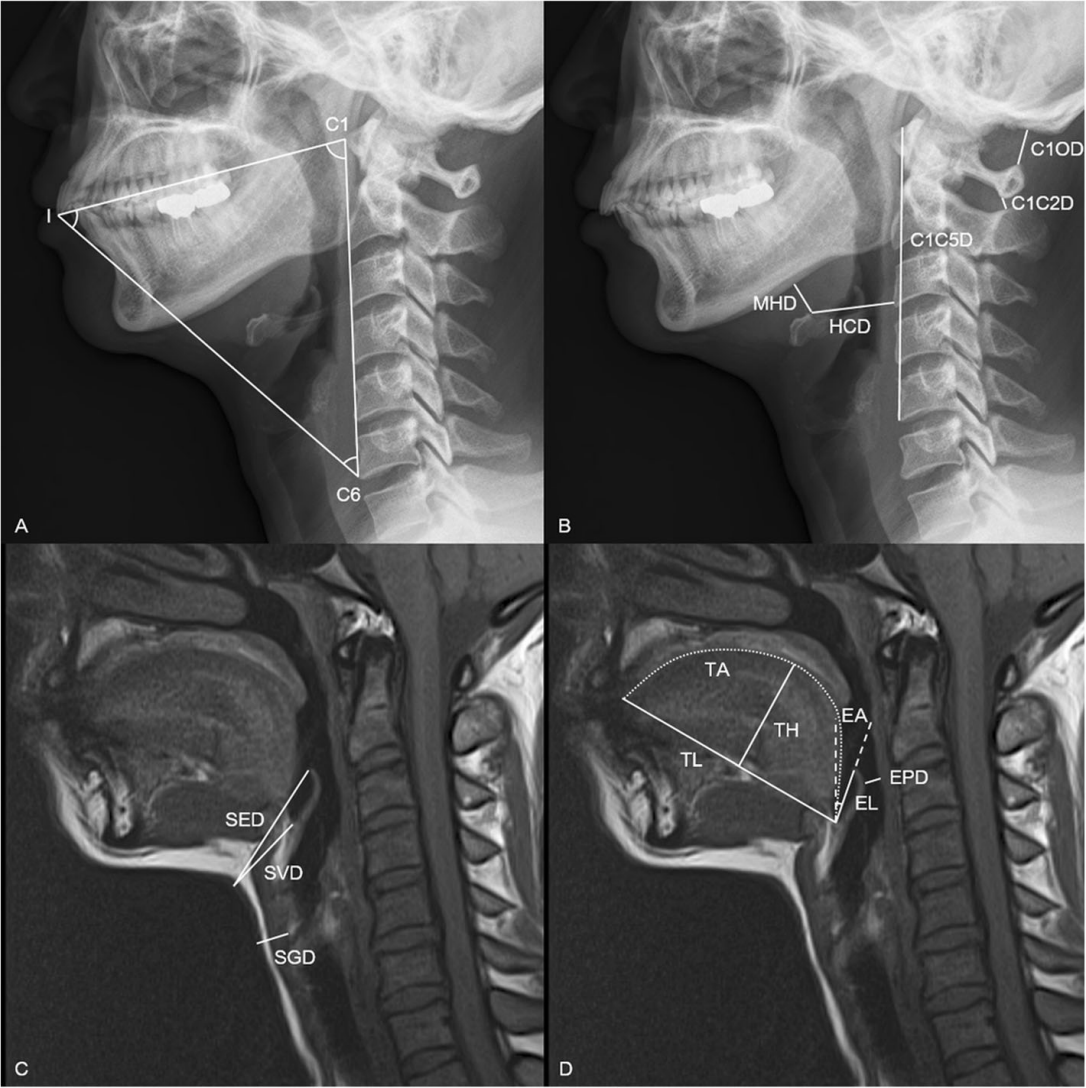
Measurements of radiographic indices investigated in this study. Radiographic indices were measured on cervical spine lateral X-ray (a and b) and magnetic resonance imaging (c and d) images in neutral neck position. I. incisor; C1, atlas; C5, the 5th cervical spine; MHD, mandibulohyoid distance; C1C5D, atlanto-the 5th cervical vertebral distance; C1OD, atlanto-occipital distance; HCD, hyoidocervical distance; C1C2D, atlanto-axial distance; SVD, skin-vallecular distance; SED, skin-epiglottic distance; SGD, skin-glottic distance; TL, tongue length; TH, tongue height; TA: tongue area; EL, epiglottis length; EPD, epiglottic-pharyngeal distance, EA, epiglottis angle
